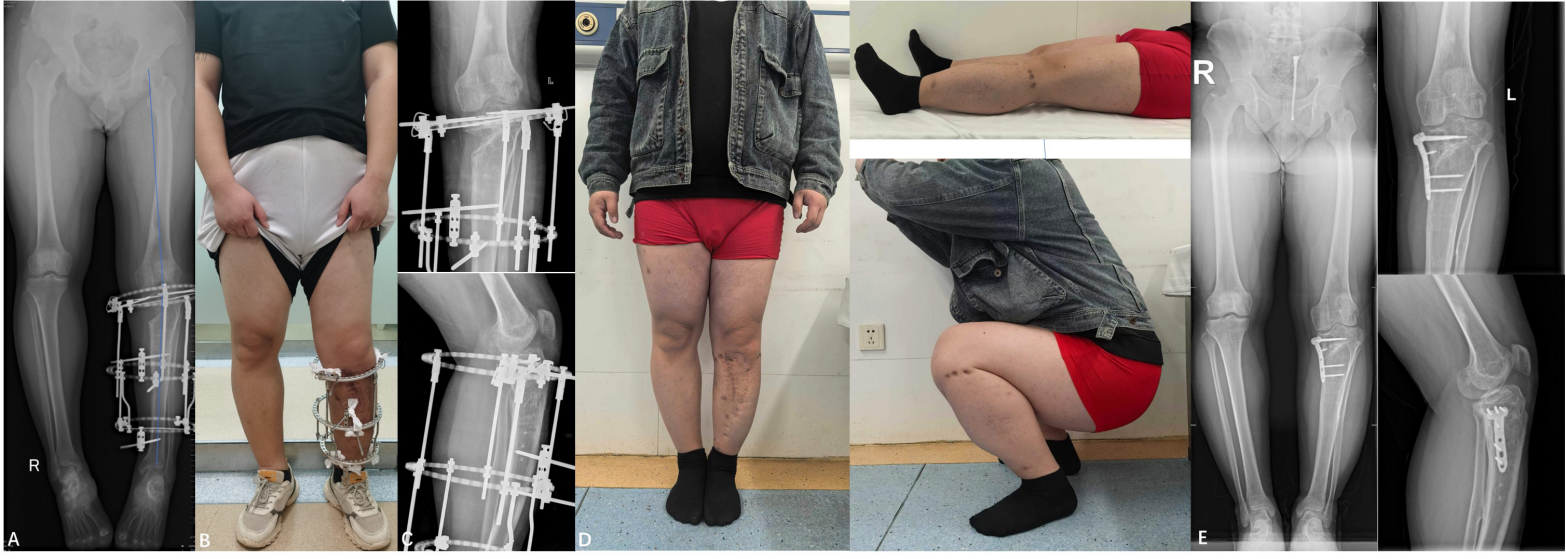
(A) Full-length X-ray of both lower limbs (April 2022, post-third surgery): Features the external fixator in a good position, normal force lines of both lower limbs, aligned osteotomy ends, the defect filled with high-density bone, and the left limb being shorter than the right by 1 cm, MAA: 176°. (B) Photograph (June 2022, post-third surgery): Shows the lower limb deformity completely corrected and fixed with an external fixator, with no obvious discomfort observed. (C) X-ray of left tibia and fibula (January 2023, post-third surgery): Demonstrates a medial bone defect in the proximal tibia, good alignment of the osteotomy ends, and a well-positioned external fixator ten months following surgery. (D) Photograph (February 2023): Image captured after the removal of the external fixator. (E) X-ray(February 2023):MAA:174°.
